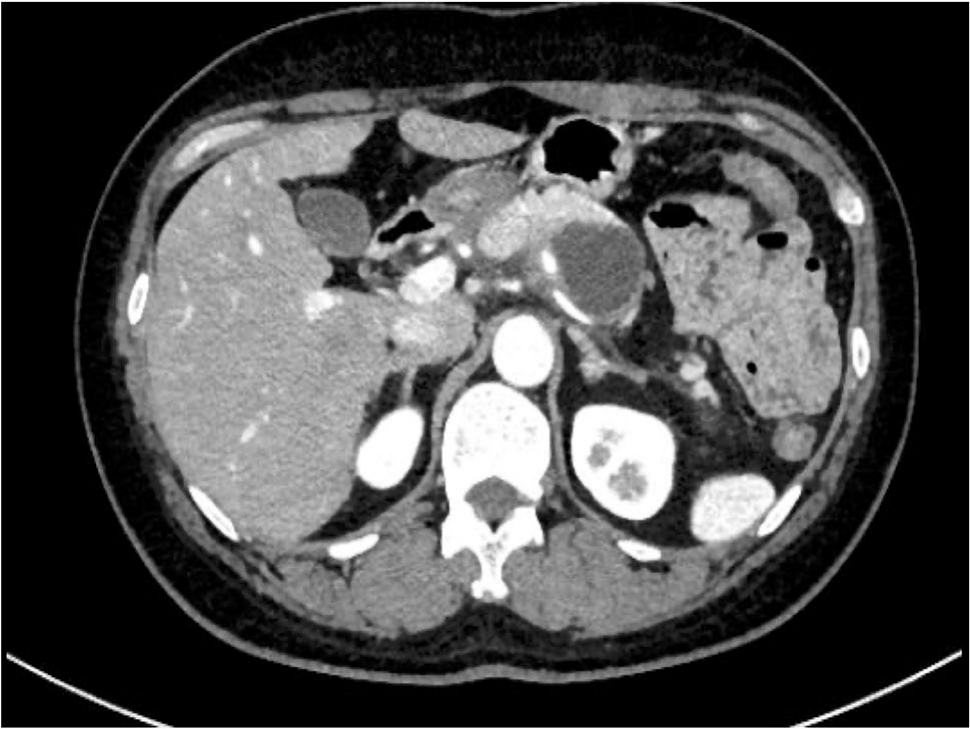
Computed tomography 3 months later showed a slight increase in cyst diameter
